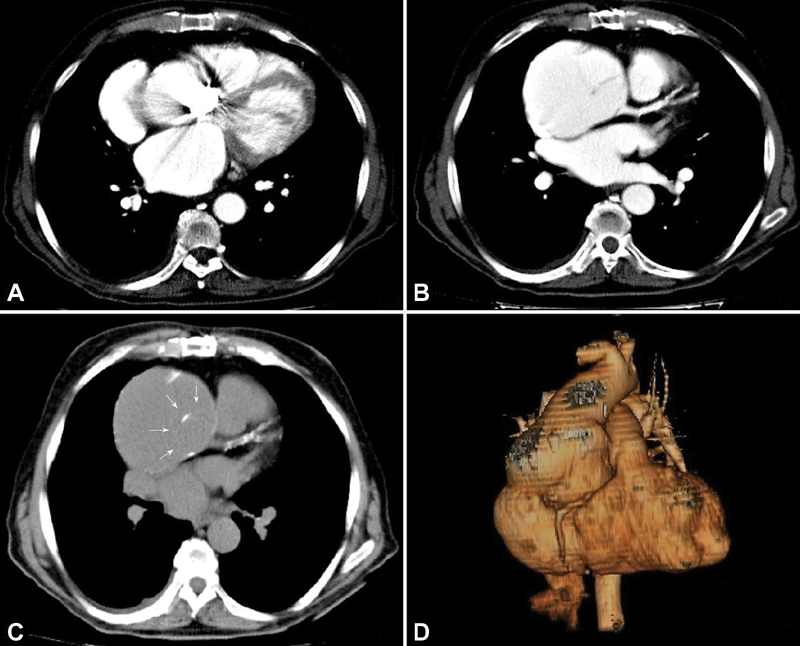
Computed tomography angiography scans revealed that both the coronary arteries (
